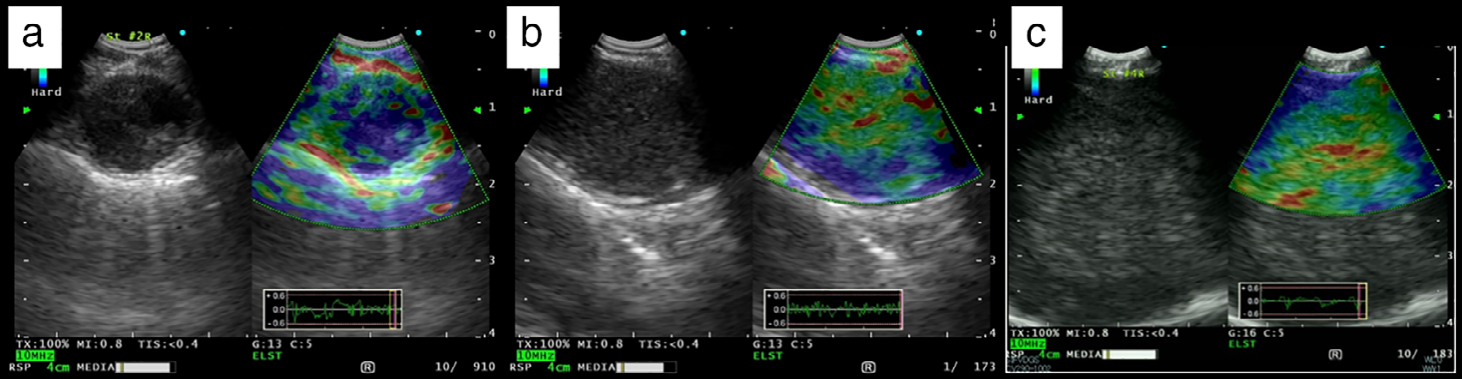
(a) Malignant lymph node pattern on elastography. The 2R lymph node was 13.6 mm in size, and abnormal FDG uptake on PET‐CT in the node indicated malignancy on elastography. The final diagnosis was malignancy. (b) Benign pattern lymph node pattern on elastography. The 3p lymph node was 17.6 mm in size, and abnormal FDG uptake on PET‐CT in the node indicated benignancy on elastography. The final diagnosis was benign. (c) False‐negative case. The 4R lymph node was 34.5 mm in size, and abnormal FDG uptake on PET‐CT in the node indicated benignancy on elastography. However, the final diagnosis was malignancy.
